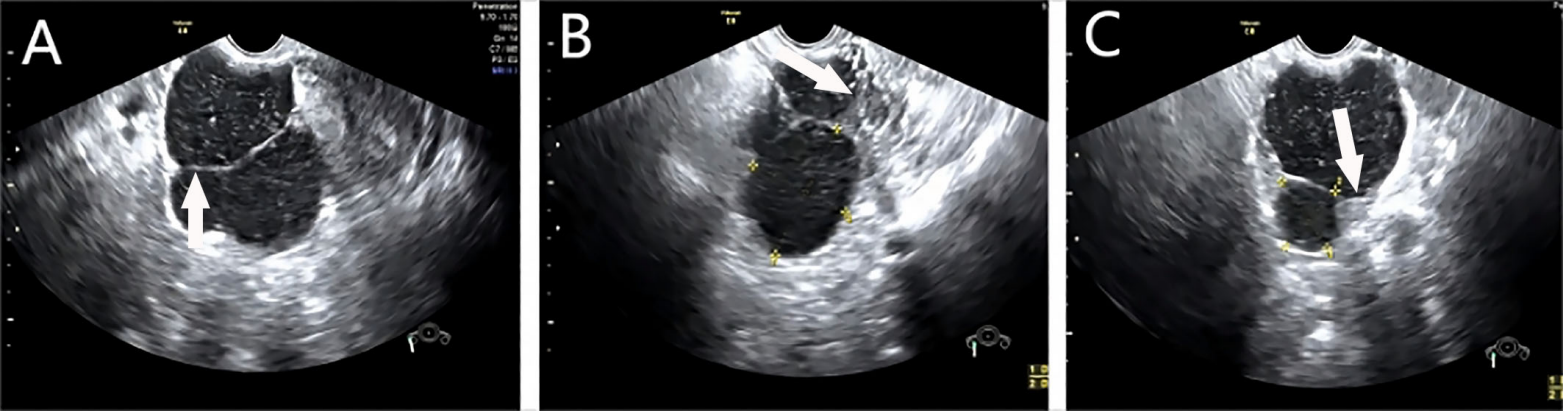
A right ovarian multilocular cystic mass measuring 13.6 x 11.8 x 10.2 cm, with multiple septations of variable thickness, focal thickening is observed in the cyst wall, poor sound transmission, and internal punctate echoes. (A) shows a transvaginal ultrasound image with a white arrow pointing to a septation within a large ovarian cyst. (B) displays another section of the cyst with an arrow indicating mural nodularity. (C) highlights a different view of the same cyst, with an arrow pointing to a thickened cyst wall.
